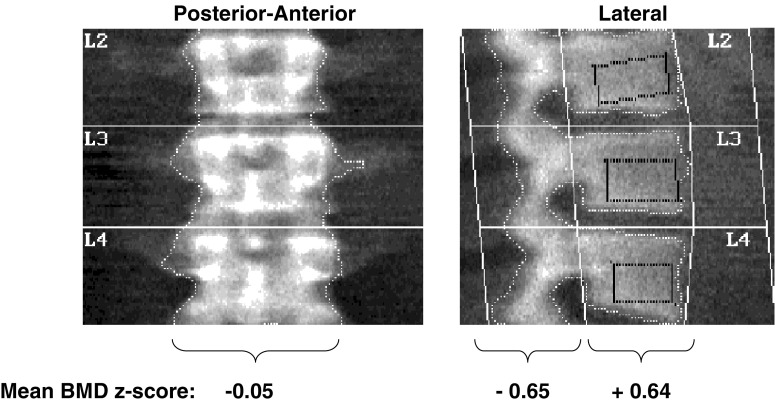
Differential effects of primary hyperparathyroidism on posterior–anterior and lateral dual-energy X-ray absorptiometry (DXA) bone mineral density (BMD) in the spine in adults (data derived from Duan et al. [47]
